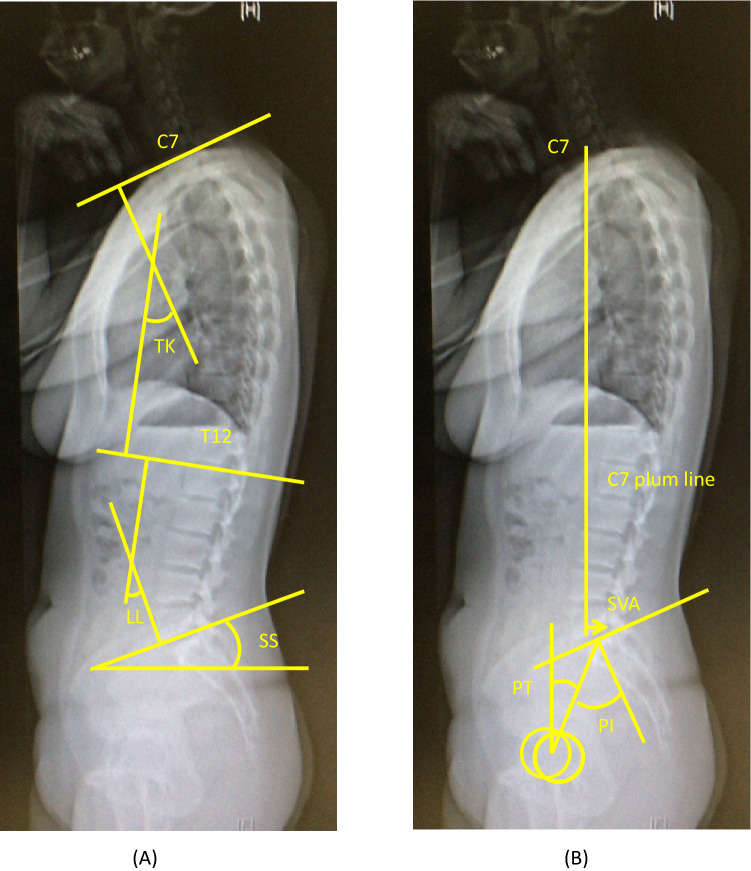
Whole spine radiographic measurements. (a) Measurement of thoracic kyphosis (TK), lumbar lordosis (LL) and sacral slope (SS). (b) Measurement of diameter of sagittal vertical axis at C7(C7-SVA), pelvic tilt (PT), and pelvic incidence (PI). Pelvic tilt (PT) is defined by the angle between the vertical and the line through the midpoint of the sacral plate to the femoral heads axis (retroversion is then measured as a pelvic tilt increase, anteversion as a pelvic tilt decreases). Sacral slope (SS) is defined as the angle between the horizontal and the sacral plate. Pelvic incidence (PI) is defined as the angle between the perpendicular to the sacral plate at its midpoint and the line connecting this point to the femoral heads axis.
